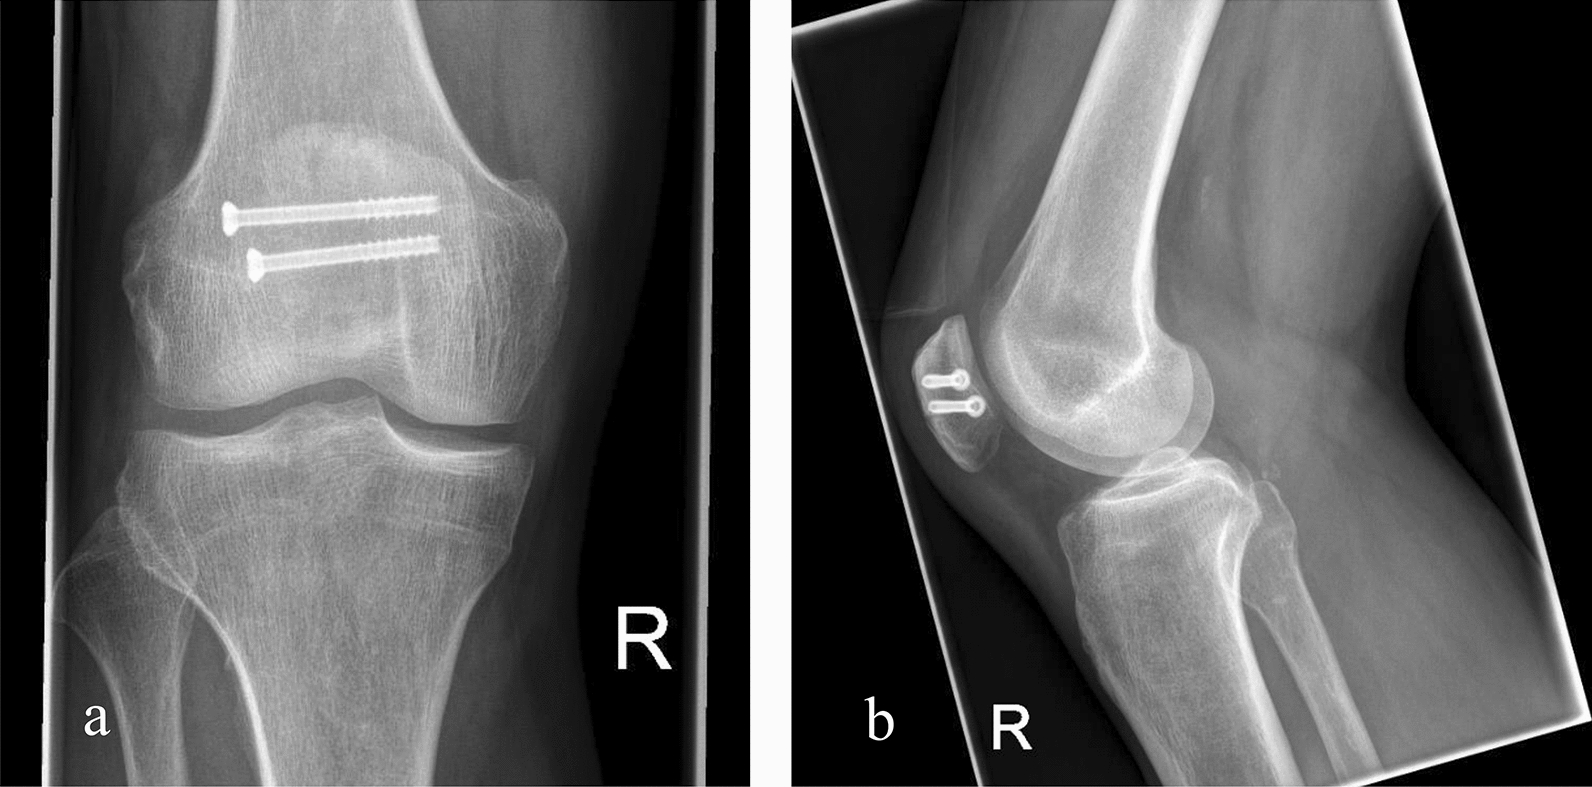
a/p (a) and lateral (b) X-ray of a simple transverse patella fracture after operative treatment using screw fixation
